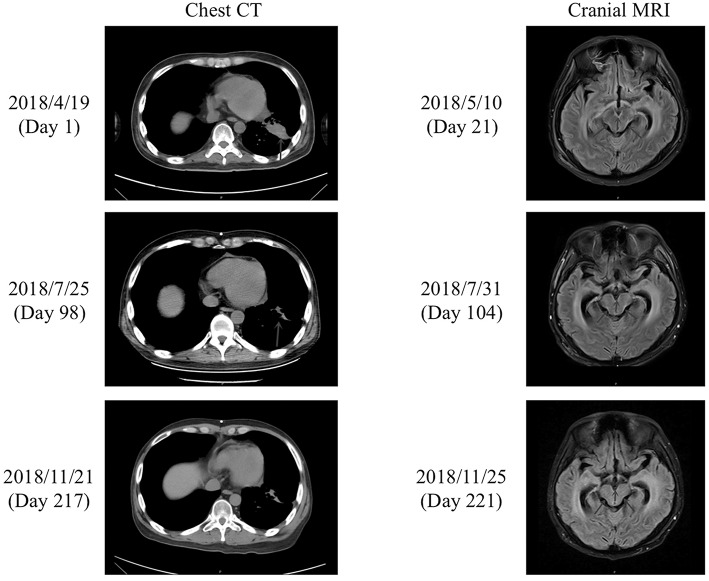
Chest CT and cranial MRI. (Left) Chest CT performed on the day of admission (day 1) and after treatment with afatinib for 71 days (day 98) and 190 days (day 217). The lung lesions (blue arrows) were reduced in size after treatment with afatinib for 71 days (day 98), and the effect was maintained after a further 119 days of treatment (day 217). (Right) Enhanced cranial MRI T2 FLAIR scans performed on day 21 and after treatment with afatinib for 77 days (day 104) and 194 days (day 221). The cranial MRI scan on day 21 showed high-intensity linear shadows in the medulla oblongata, pons, and ventral and dorsal midbrain, which were considered to be leptomeningeal metastases. The cranial scan on day 104 revealed similar abnormalities to those detected on day 21. However, the abnormal signals in the medulla oblongata, pons, and ventral and dorsal midbrain on day 221 were decreased in comparison to those on days 21 and 104.
